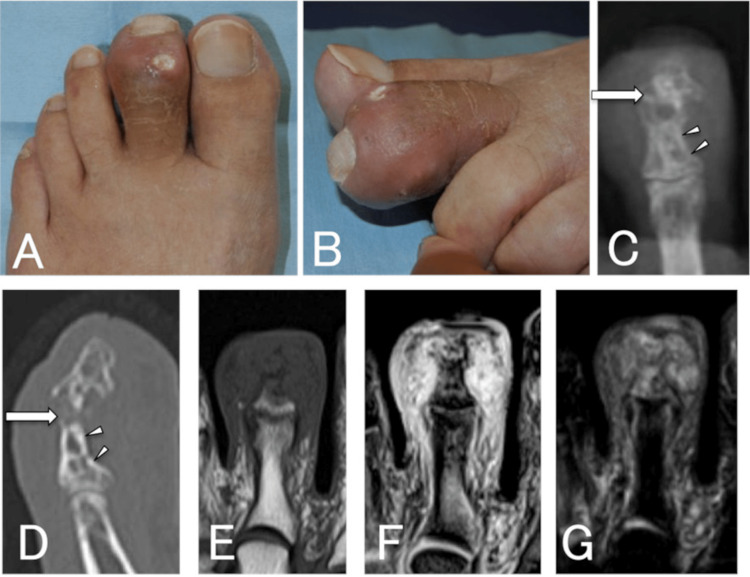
Case one: clinical and radiological findings. A, B: Photographs showing swelling of the left second toe, and powdery white contents from the ulcer exposed on the dorsal side and seen through the skin on the lateral side. C, D: A radiograph and computed tomography scan showing a fracture of the distal interphalangeal joint (arrow) and depression of the metaphysis due to the compression of the mass (arrowheads). E-G: Magnetic resonance images showing a soft tissue mass surrounding the distal and middle phalanges with a low signal on a T1-weighted image (E), a high signal on a T2-weighted image (F), and a high signal on a short tau inversion recovery image (G).
